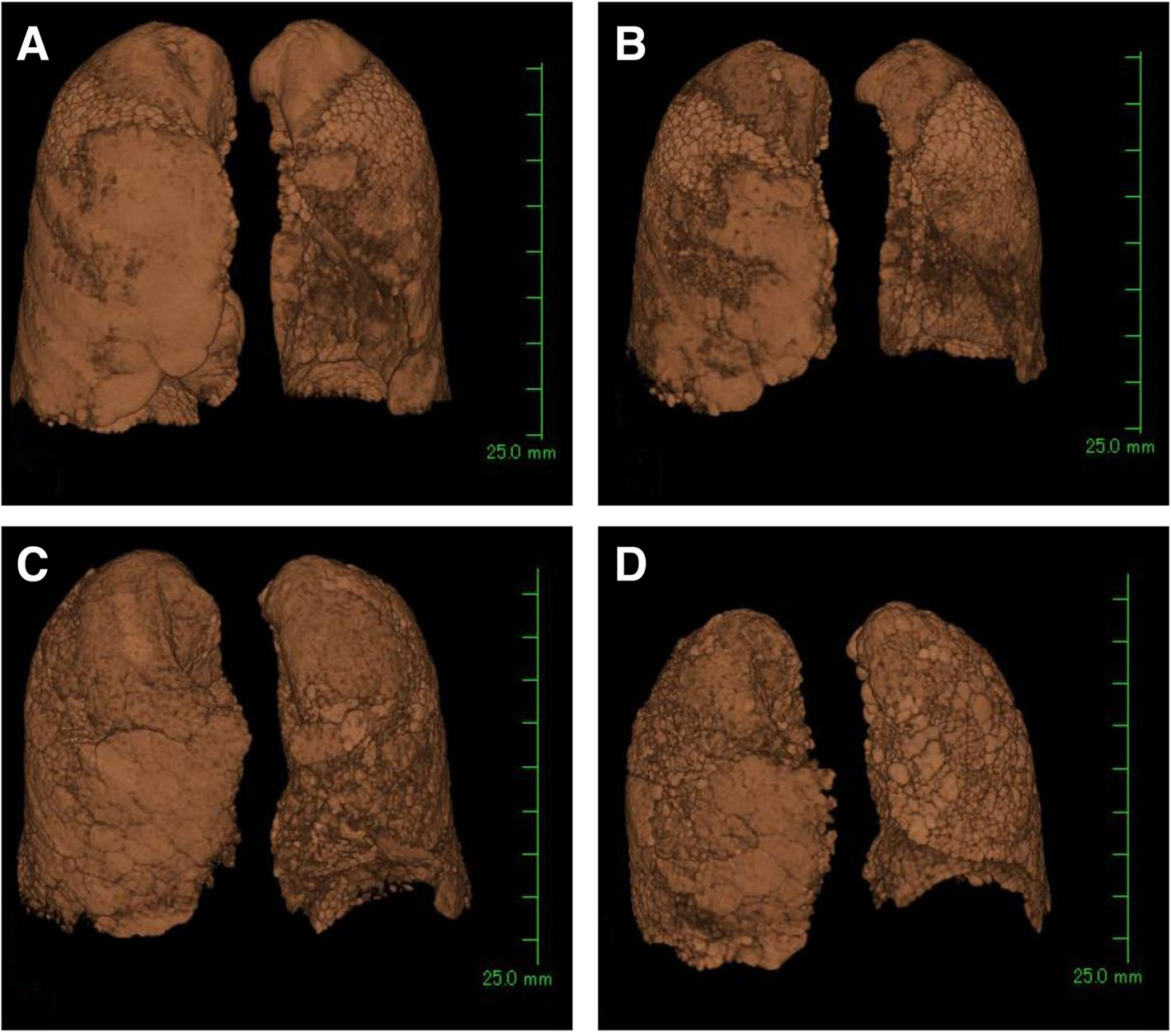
Representative data of three-dimensional reconstruction images of the lung from the CT data at the initial and following examinations. (a, b) ANCA nephritis with a UIP pattern. (c, d) IPF. (a, c) Whole-lung images reconstructed from the data of the initial CT examination. (b) CT image 48 months after the initial CT examination. (d) CT image 36 months after the initial CT examination. CT, computed tomography; ANCA, anti-neutrophil cytoplasmic antibody; UIP, usual interstitial pneumonia; IPF, idiopathic pulmonary fibrosis
